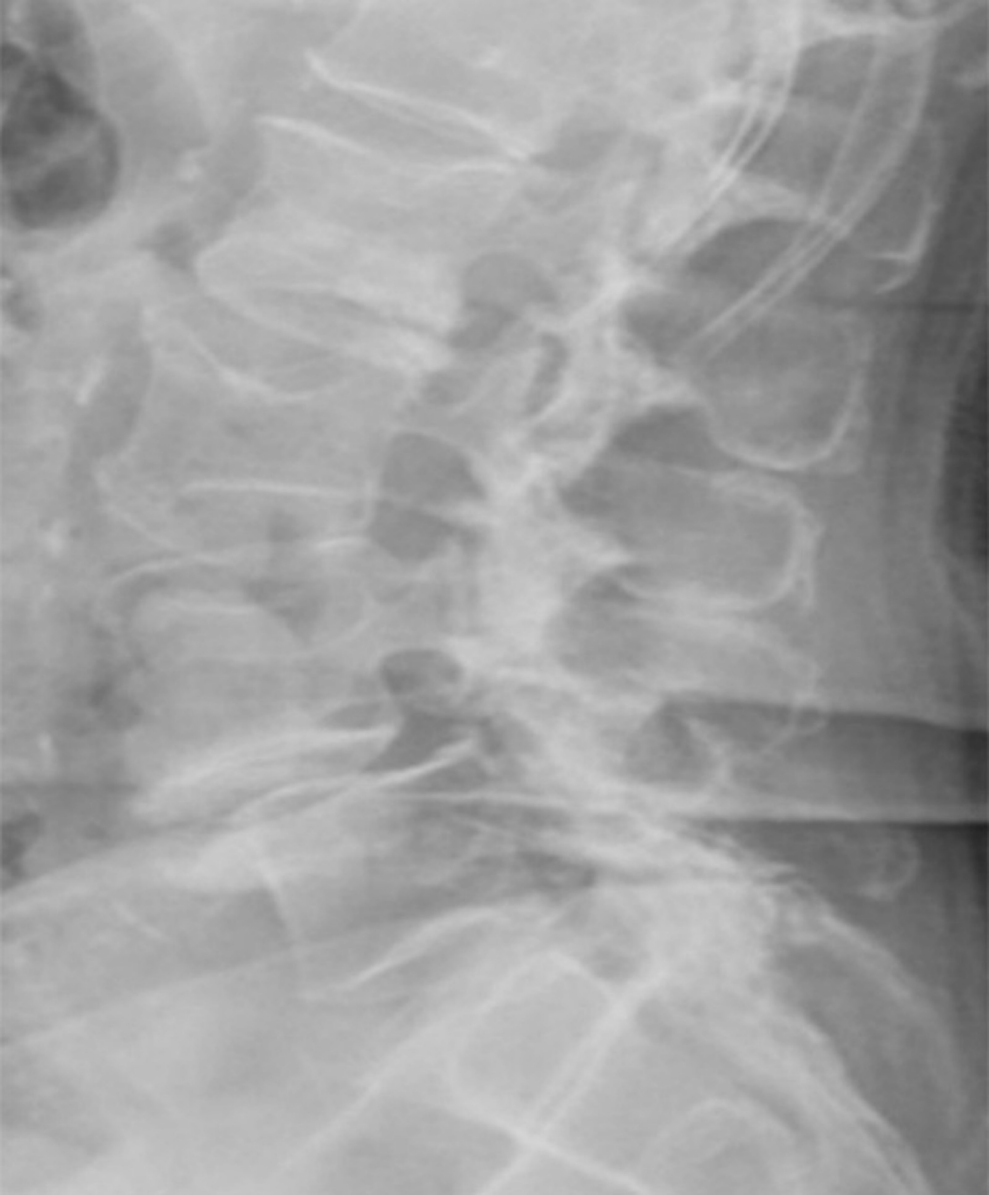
X-ray of Poor Candidate Lateral x-ray shows osteopenia/osteoporosis, which can result in interbody subsidence and need for concomitant direct decompression procedures.
